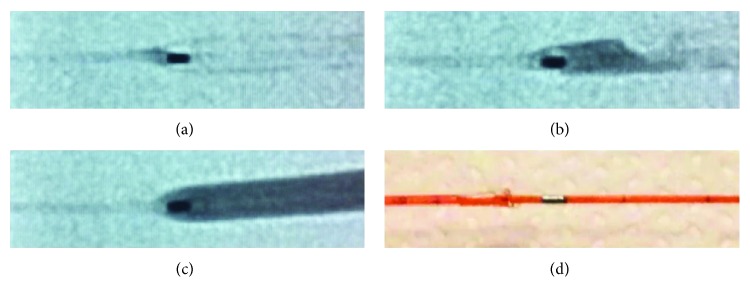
Exit/Entrance port of contrast media. (a), (b), and (c) show entrance of contrast media filling the angioplasty balloon by X-ray. (d) Photograph of a balloon catheter with the balloon cut from the rest of the catheter.
